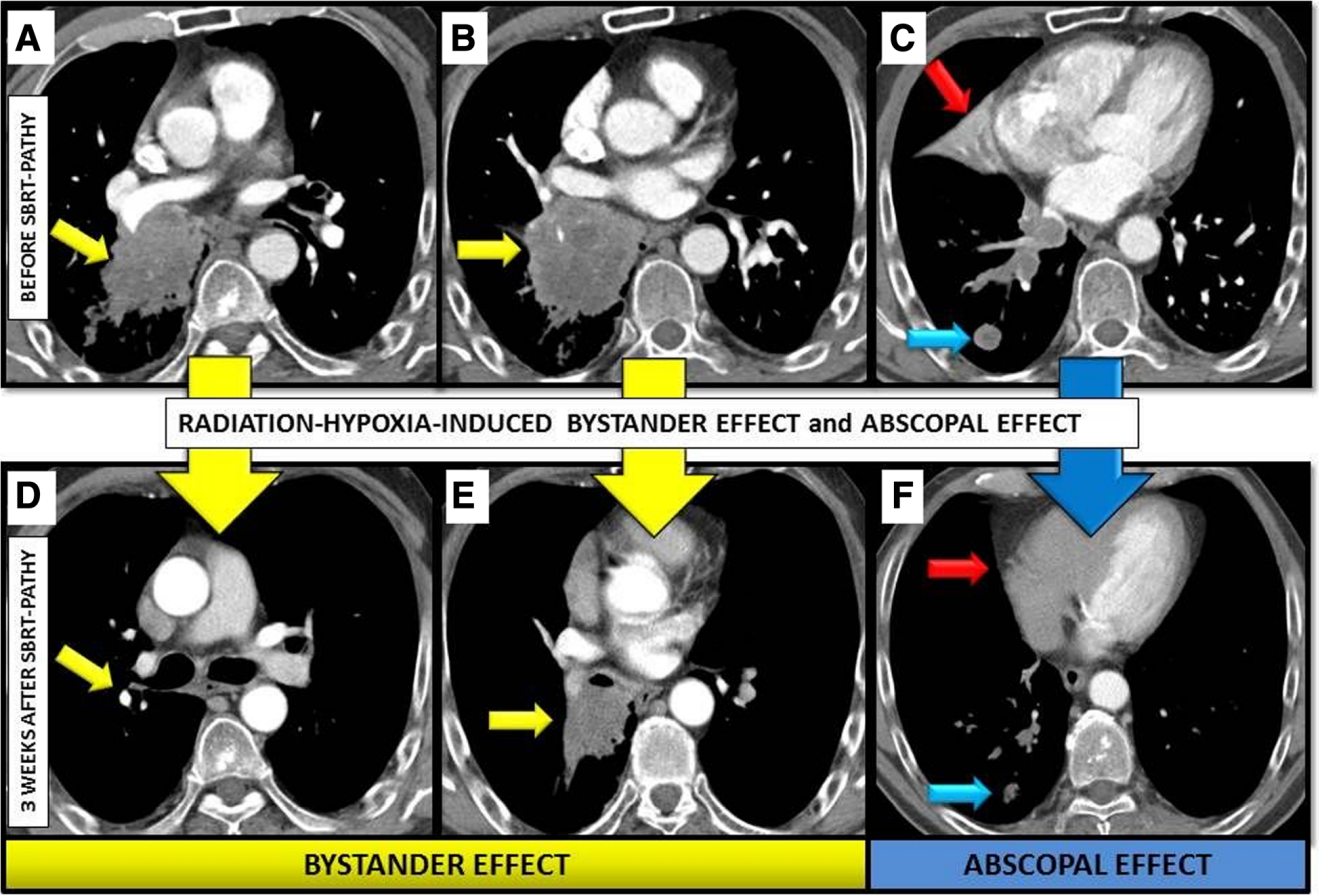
NEOADJUVANT SBRT-PATHY: a-c) diagnostic CT of the patient with unresectable squamous-cell lung cancer (yellow arrows), separate lung lesion (blue arrow), and an atelectasis (red arrow). 3 weeks after SBRT-PATHY, preoperative restaging CT (d-f) showed a 60% reduction of partially treated tumor (bystander effect: yellow arrows), a 50% reduction of unirradiated lung lesion (abscopal effect: blue arrows), and complete regression of atelectasis (red arrows)
